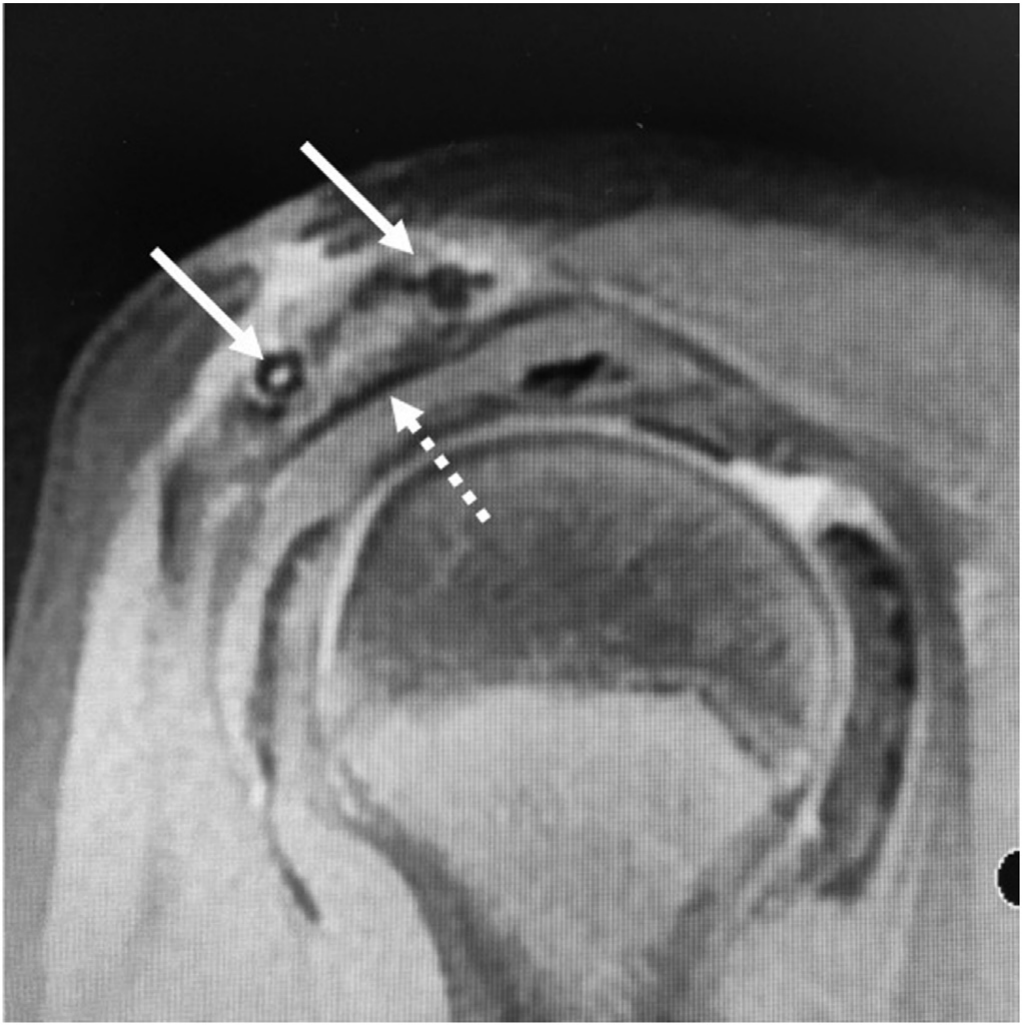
Three-month postoperative coronal plane MRI, showing well-positioned screws and physeal ossification (arrows) with bone bridging between the epiphysis and metaphysis (dotted arrow). *MRI*, magnetic resonance imaging.
